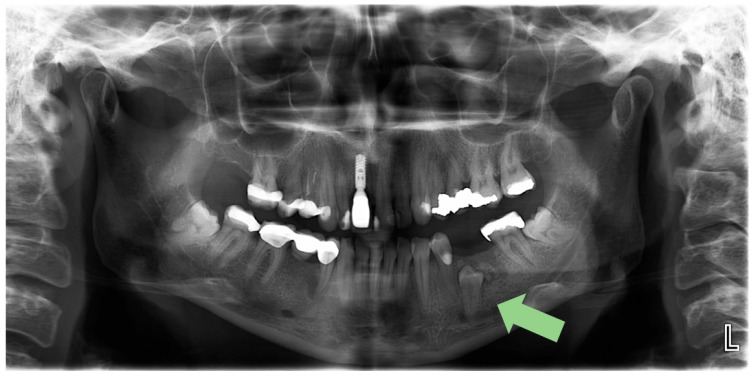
Orthopantomogram (OPG) of the patient and the arrow indicates the impacted mandibular left second premolar.
